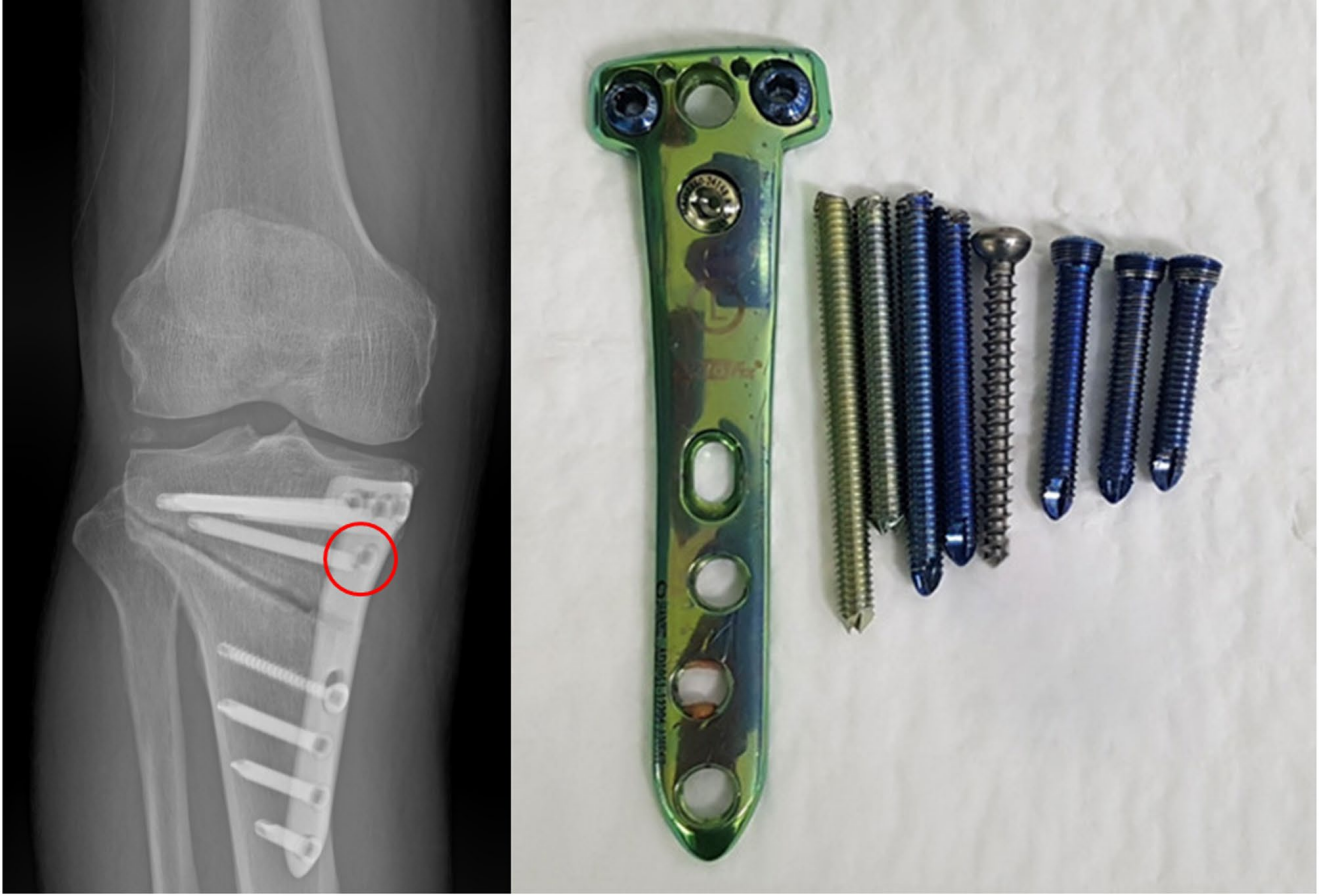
Locking screw breakage at the head and neck junction in opening-wedge high tibial osteotomy. Postoperative radiograph showing fixation with locking plate and screw. The red circle indicates definite screw breakage. Removed hardware components
